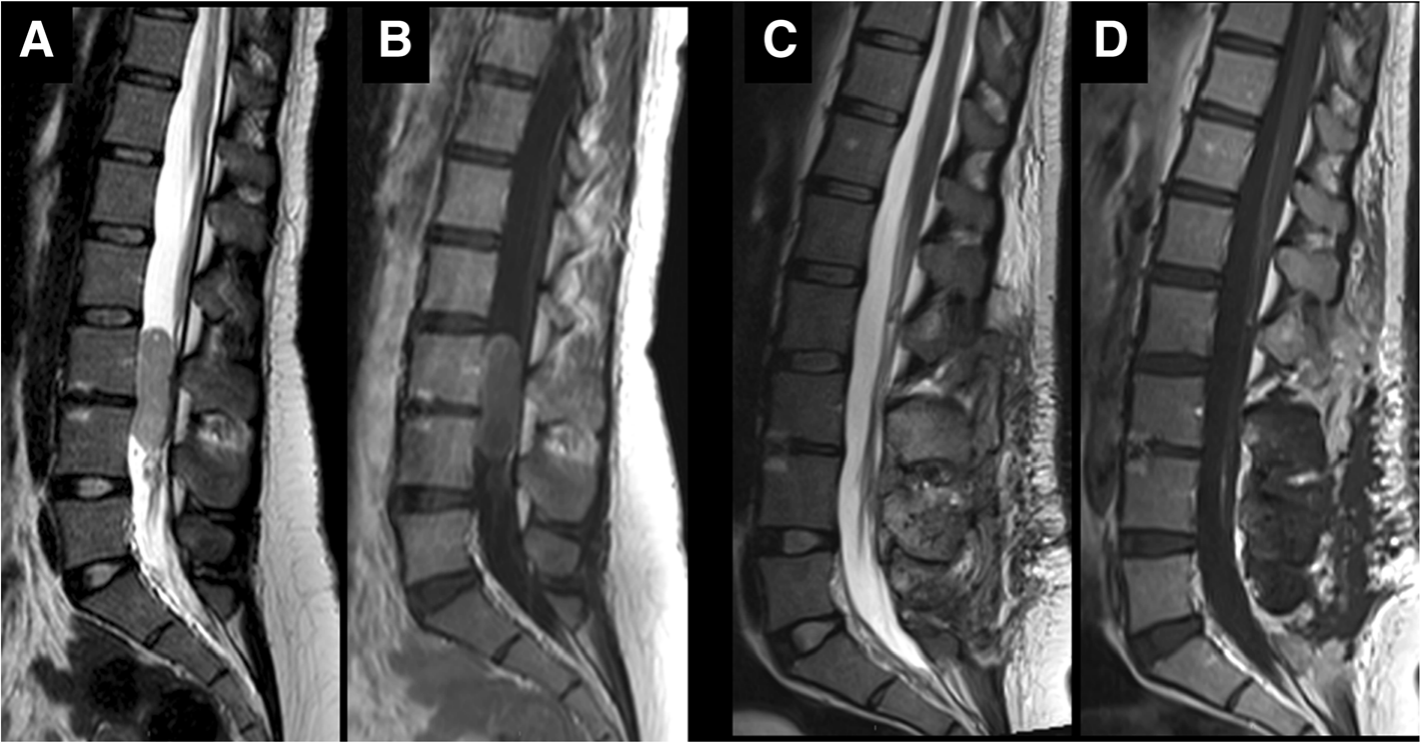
Spine MRI, sagittal pre-operative and post-operative images. Sagittal pre-operative TSE T2 WI (a) and T1 WI with Gd (b) show a lumbar endocanalar mass extending from L3 to L4, isointense on T1 and T2 WI with peripheral contrast enhancement. Sagittal post-operative TSE T2 WI (c) and T1 WI with Gd (d) show total resection of the tumor
